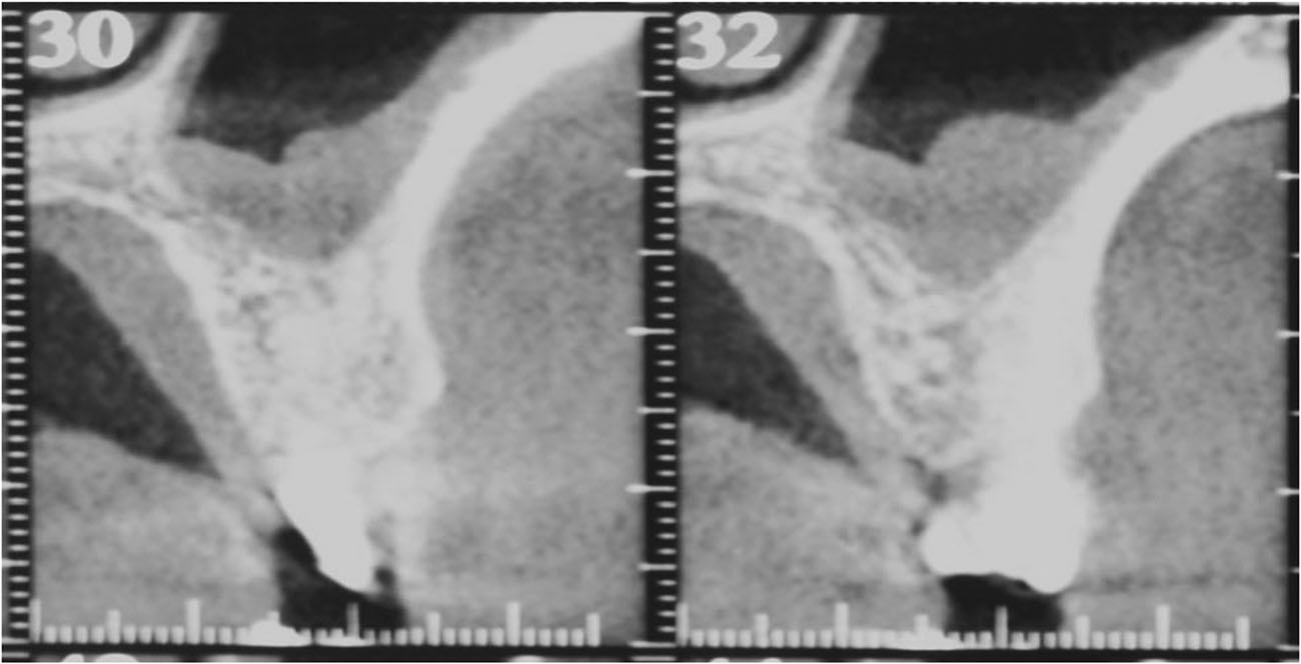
Presurgical cone beam computed tomography
